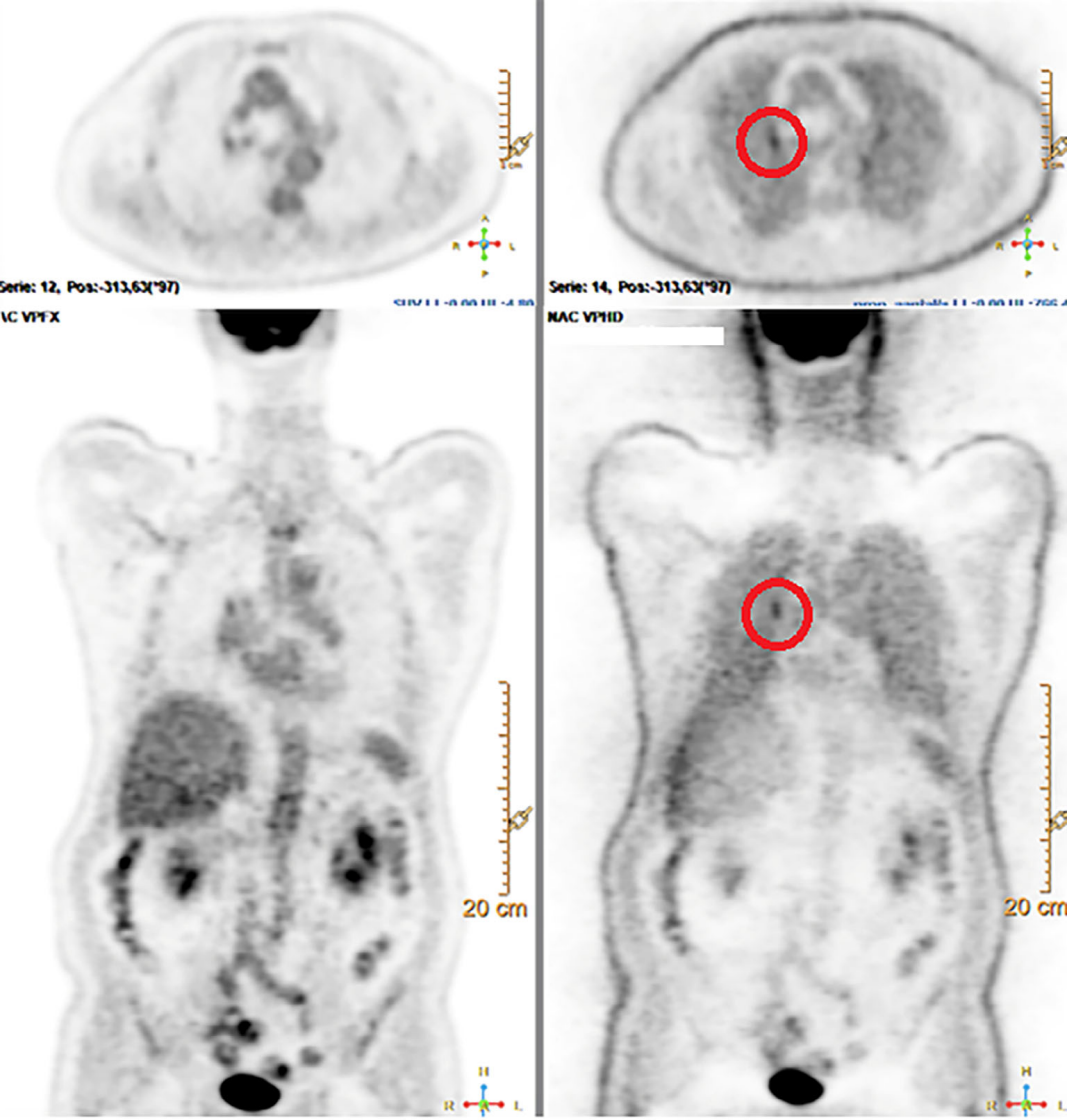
Example of axial and coronal view of CTAC PET on the left and NAC PET on the right of a patient with a pre‐invasive lesion in the right upper lobe that was initially missed on the CTAC images. CTAC, computed tomography‐based attenuation‐corrected; PET, positron emission tomography; NAC, non‐attenuation‐corrected.
